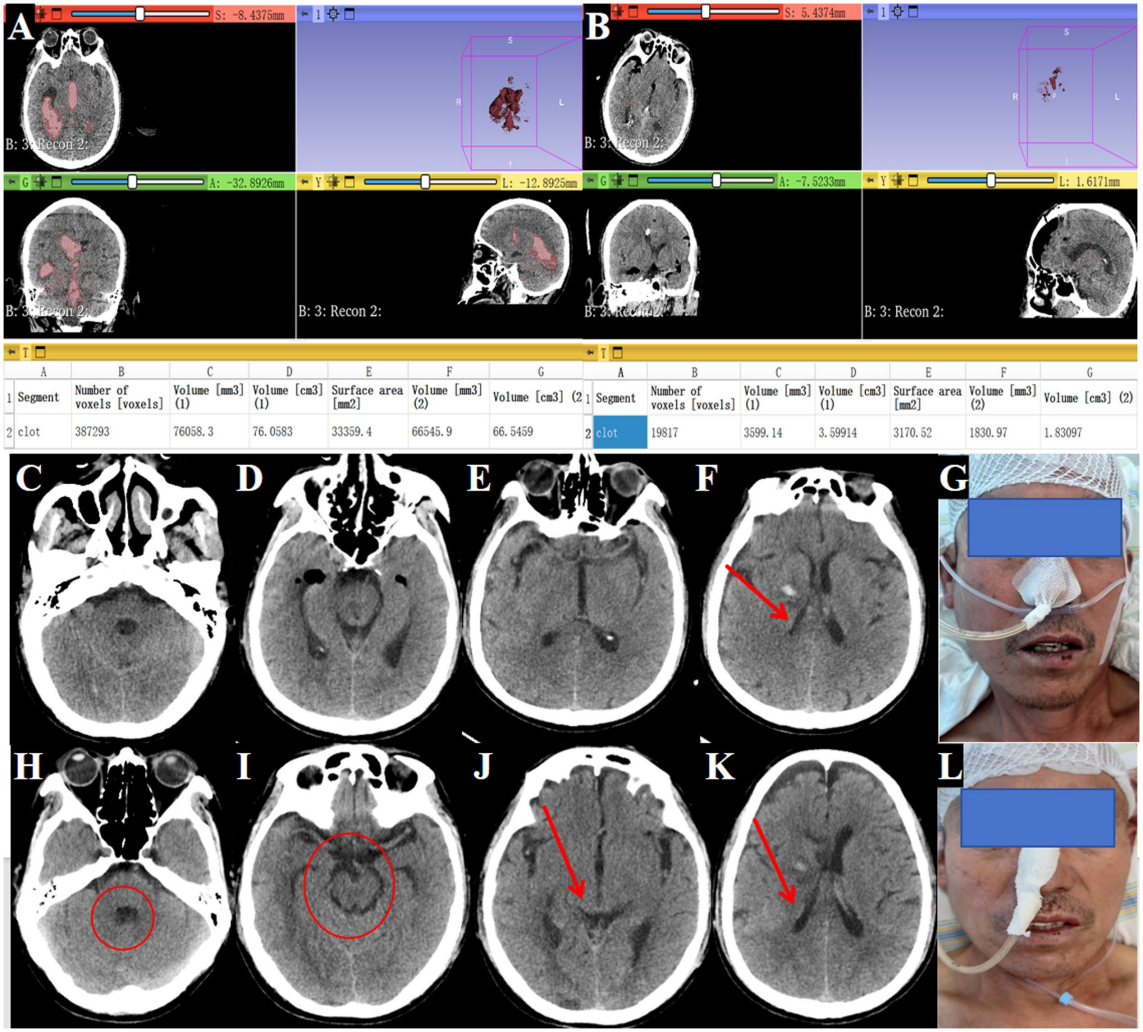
Postoperative improvement in consciousness and outcomes. (A) Preoperative head CT showing hematoma volume of 76.1 mL (→ arrows mark hemorrhage in lateral, third, and fourth ventricles). (B) Postoperative head CT showing hematoma volume reduced to 3.6 mL (→ arrows indicate evacuated regions; ◯ circles highlight restored ventricular patency). (C–F) Drainage tube removal on postoperative day 3 (→ arrow marks tube site). (G) Patient with improved consciousness responsiveness shown in clinical image. (H–K) Tenth postoperative day CT showing no hydrocephalus (→ arrows denote normalized ventricular size; ◯ circles confirm absence of residual clots). (L) The patient was alert and neurologically stable.
